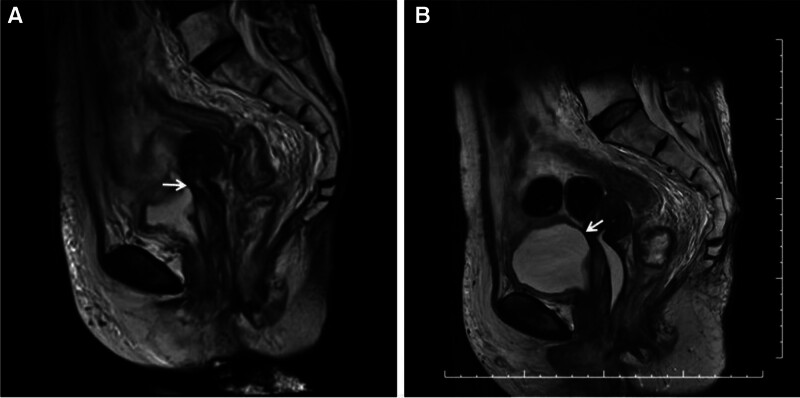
MVF MRI after fourth brachytherapy treatment. (A) MVF closure observed at the completion of brachytherapy, White arrow indicated the site of MVF; (B) MVF closure observed 1 month after the fourth brachytherapy treatment. White arrow indicated the site of MVF. MRI = magnetic resonance imaging, MVF = malignant vesicovaginal fistula.
